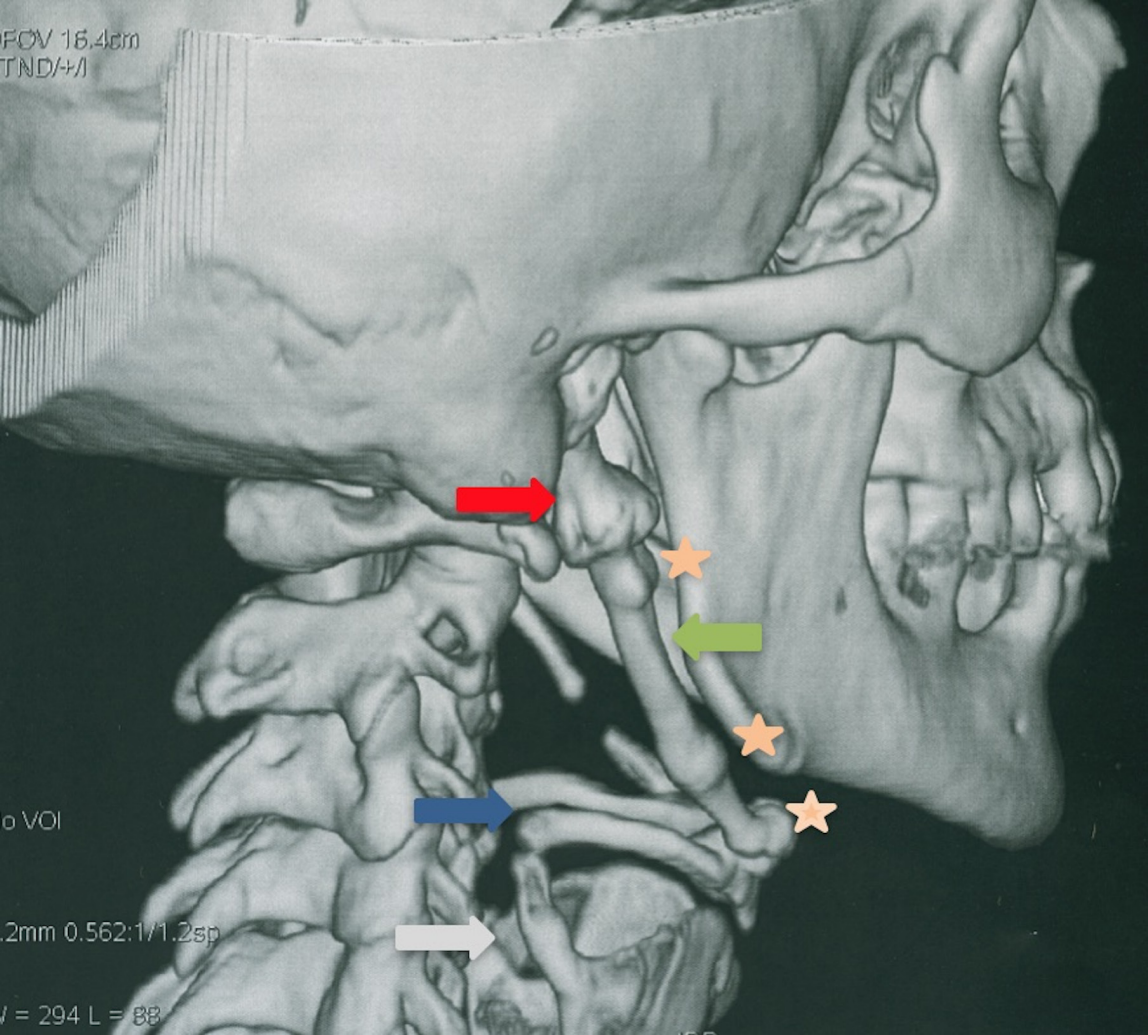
CT scan (3D VRT) of the neck shows complete ossified styloid chain and thyrohyoid ligament.
